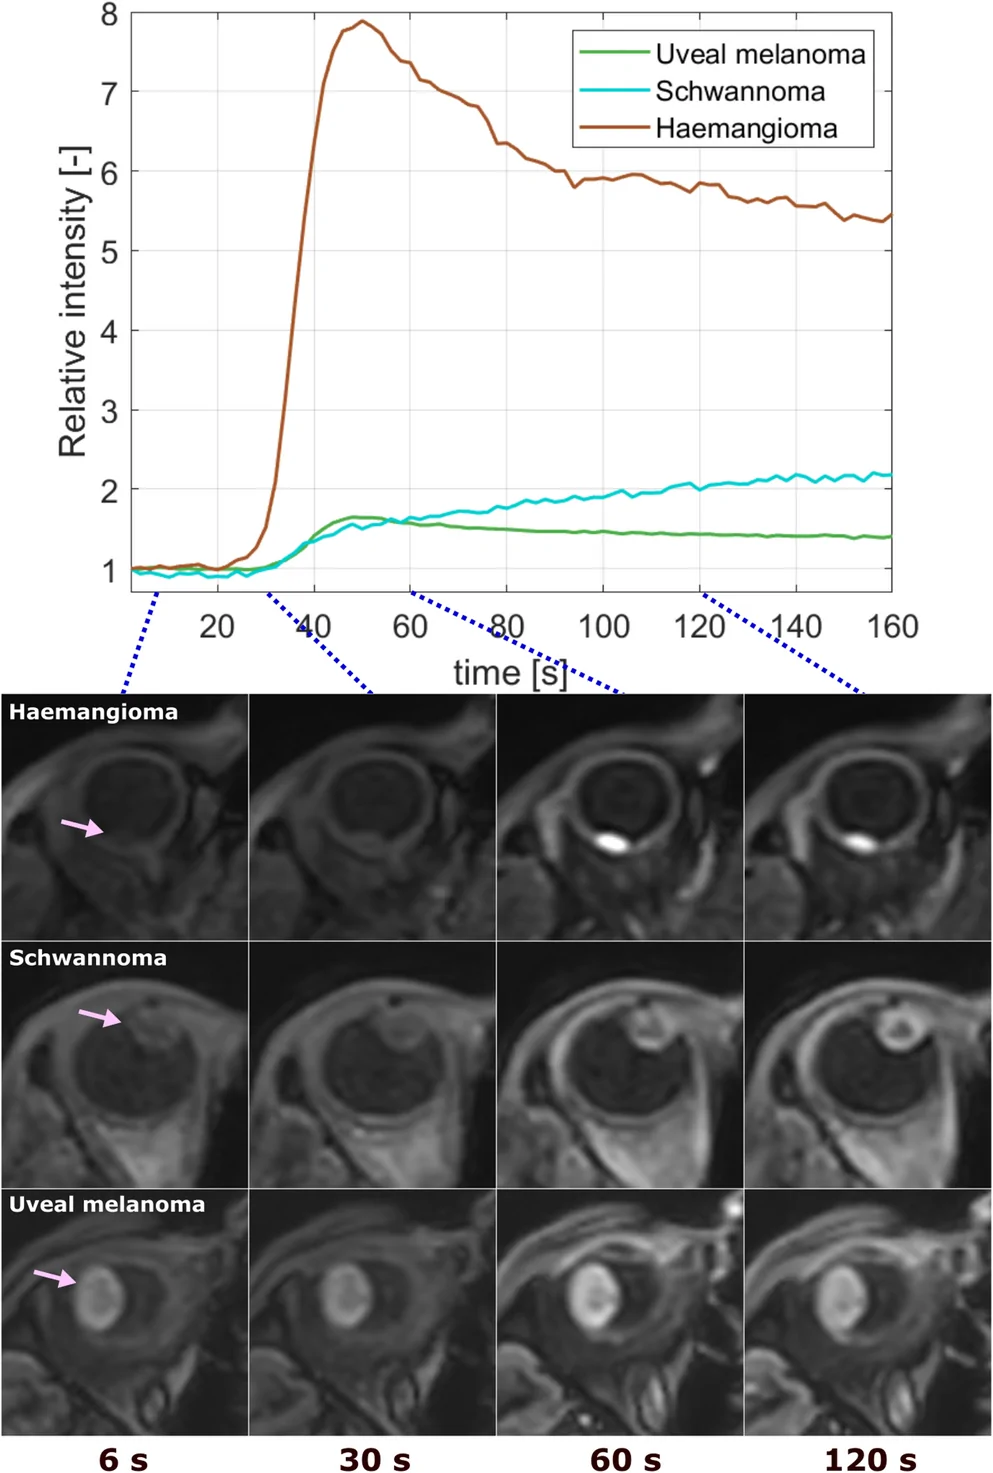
Exemplary TIC of a haemangioma (patient 03), schwannoma (patient 26), and uveal melanoma (patient 28), along with the DCE-MRI at 6, 30, 60 and 120 seconds after the bolus arrival time. More information on individual patients can be found in Supplementary Tables 1 and 2
